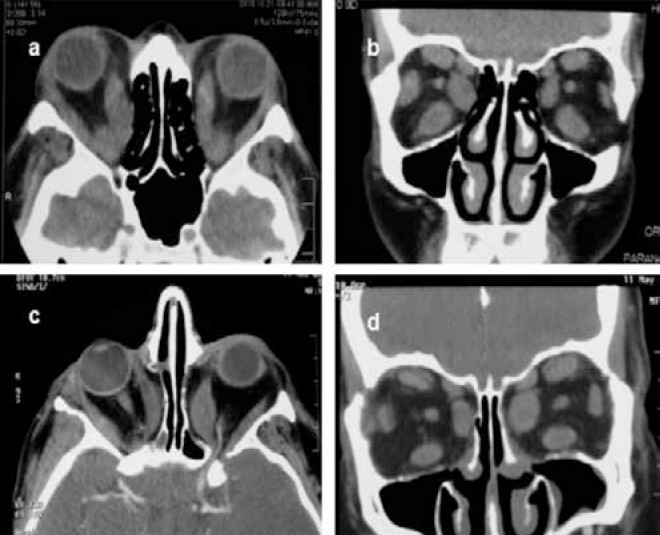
Orbital computed tomography images of a patient before (a, b) and after (c, d) decompression surgery. Proptosis was reduced in both eyes postoperatively
